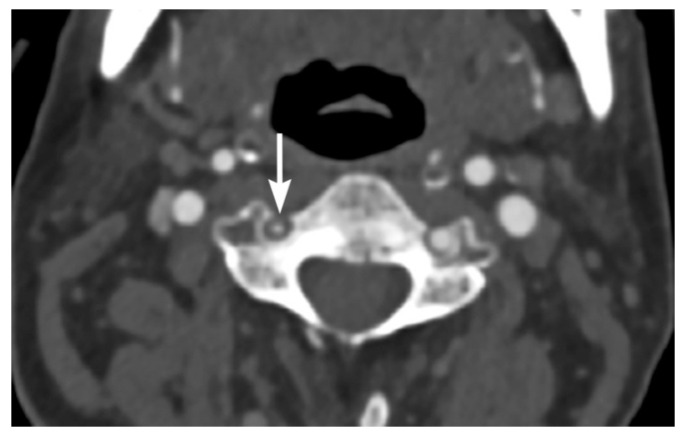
40-year-old female with right vertebral artery intramural hematoma. Axial image multidetector CT angiographic image shows right vertebral artery mural thickening consistent with intramural hematoma with moderate luminal narrowing.
